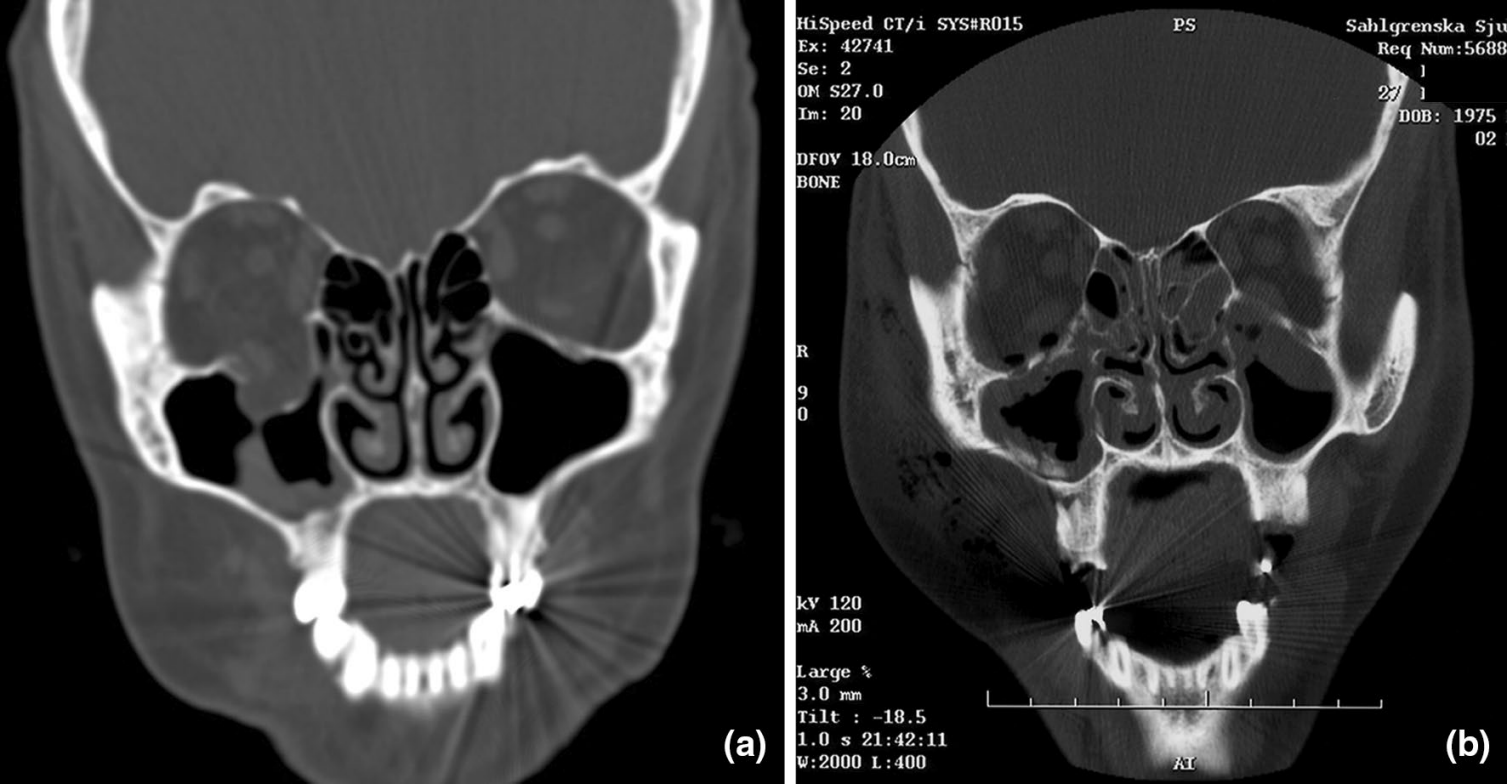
a, b Coronal CT scans. a Orbital floor defect with herniation of orbital soft tissues (right side), b “egg shell fracture” of orbital floor (right side)
